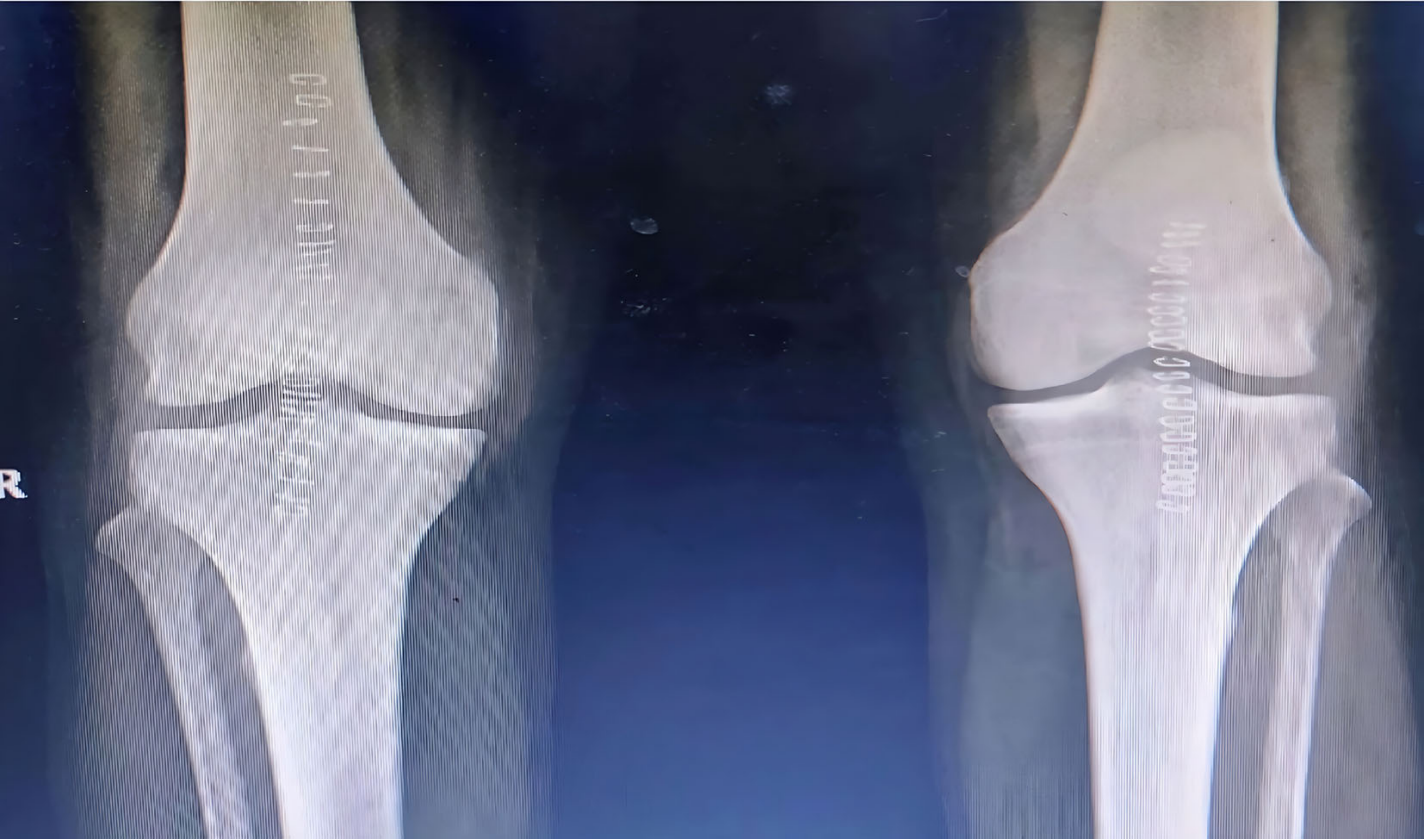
Post operative radiographs of 3 after patellar tendon repair.
